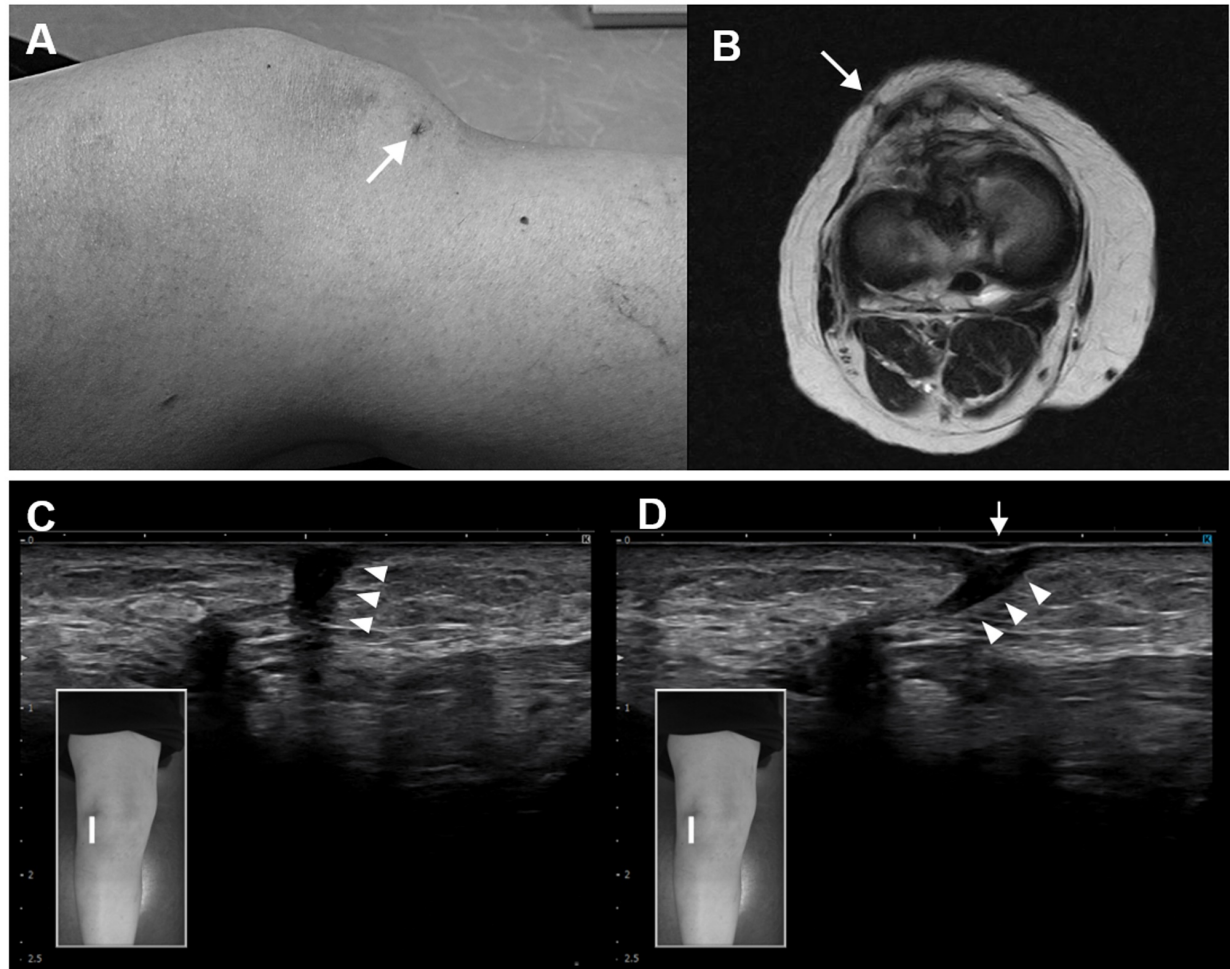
Postoperative scarring (A) Exterior view of the right knee -- scar on the patient’s right knee (arrow). (B) Magnetic resonance imaging -- scar of the skin incision from the previous arthroscopic surgery (arrow). (C) Ultrasound imaging -- hypoechoic in the scar area (arrowhead). (D) The scar area is not sliding (arrowhead) with manual sliding of the skin. The surface of the skin is deeply retracted and concaved (arrow).
